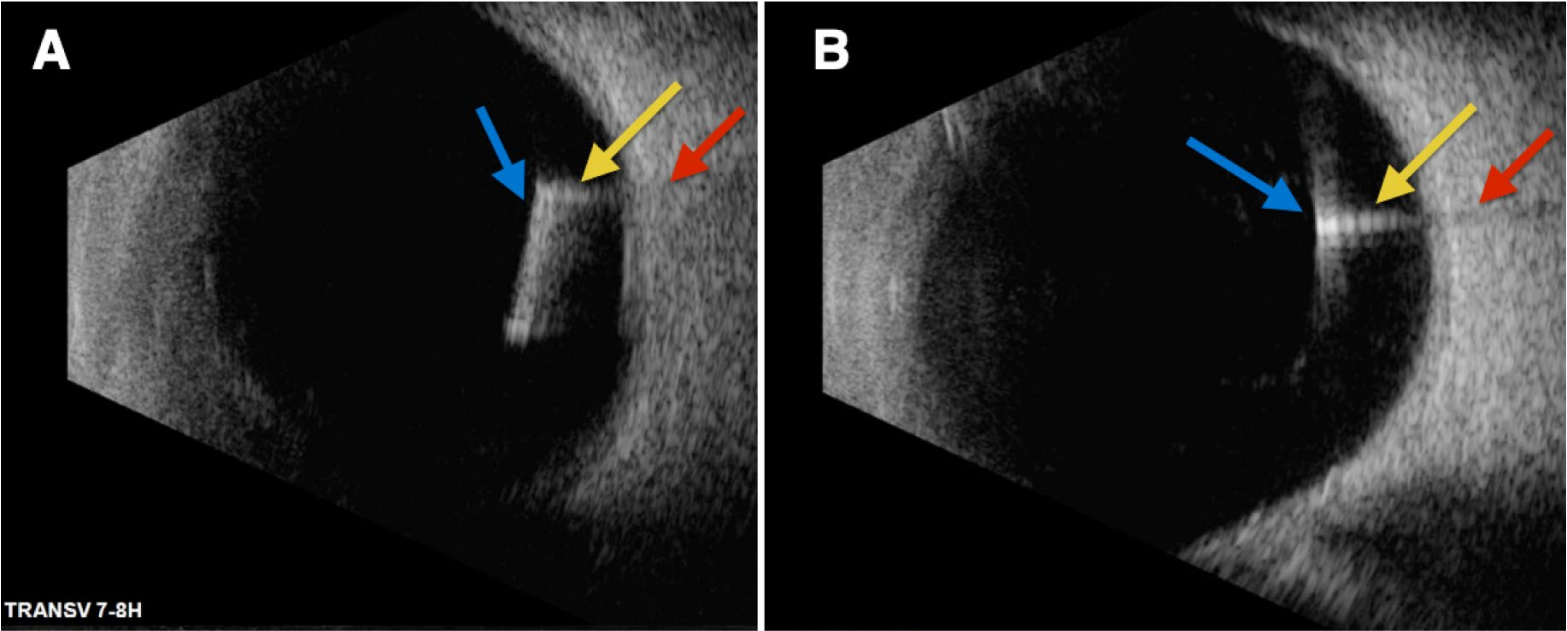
Ultrasound B-scan images of the same Ozurdex^®^ implant (blue arrows) in D0 visit, on total extension (A) and segmented (B). It is possible to evidence artifacts of reverberation in the vitreous cavity (yellow arrows) and shadowing of the eye wall (red arrows)
